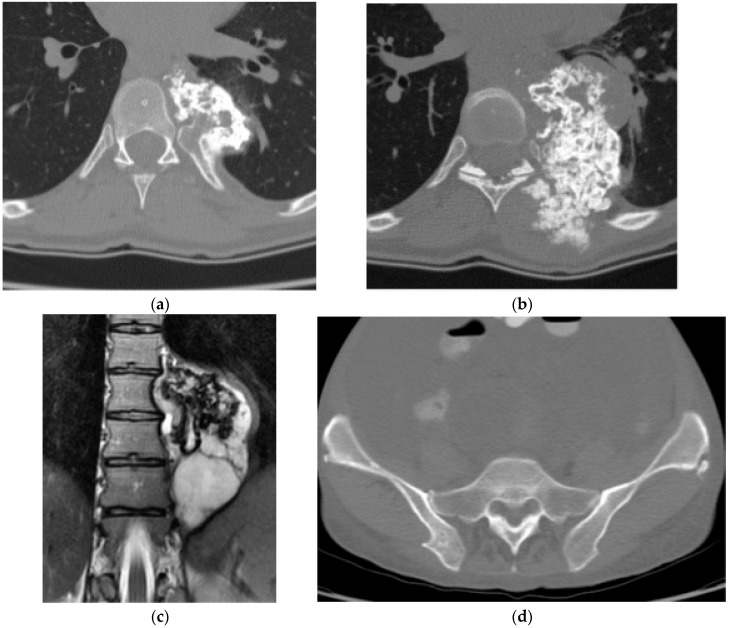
Osteochondroma with malignant, sarcomatous transformation. (a) Axial CT bone window, pedunculated osseous mass in a patient with multiple hereditary exostoses (MHE) with medullary cavity and cortex that is congruent with the normal adjacent bone. (b) Extensive calcifications are present. (c) Coronal T2 MR: low T2 signal indicates the calcification seen on CT. Large cystic component is present likely representing necrosis. (d) Small osteochondroma is present in the right iliac bone in this same patient with MHE.
